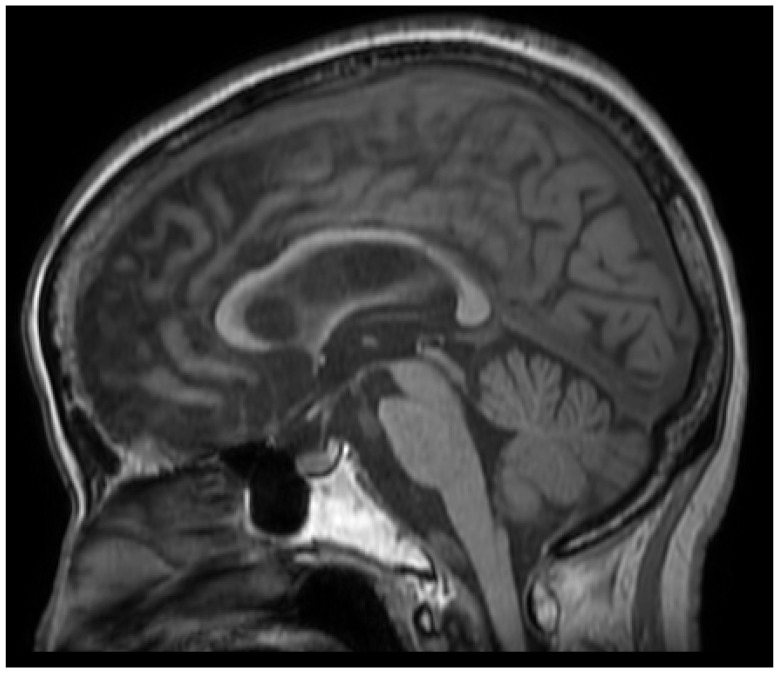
Brain MRI on T1-weighted imaging results. No atrophy of the brainstem or cerebellum, including the midbrain tegmentum, was observed. To objectively evaluate the clinical Parkinsonism as well as hyperreflexia observed in this case, the magnetic resonance Parkinsonism index (MRPI) was calculated. The MRPI is a robust quantitative imaging marker that is used to differentiate progressive supranuclear palsy (PSP) from Parkinson’s disease and other Parkinsonian syndromes by measuring the area of the pons and midbrain, and the width of the middle and superior cerebellar peduncles [10,11]. While an MRPI value of ≥12.6 is highly sensitive and specific for a diagnosis of PSP [10,11], the MRPI in our patient was 1.79. This significantly low value, along with the preserved midbrain and superior cerebellar peduncles, helps distinguish the patient’s Parkinsonian features from the structural changes typical of classic PSP, supporting the interpretation that these clinical signs may represent a distinct phenotype or an associated phenomenon rather than primary midbrain degeneration [10,11].
